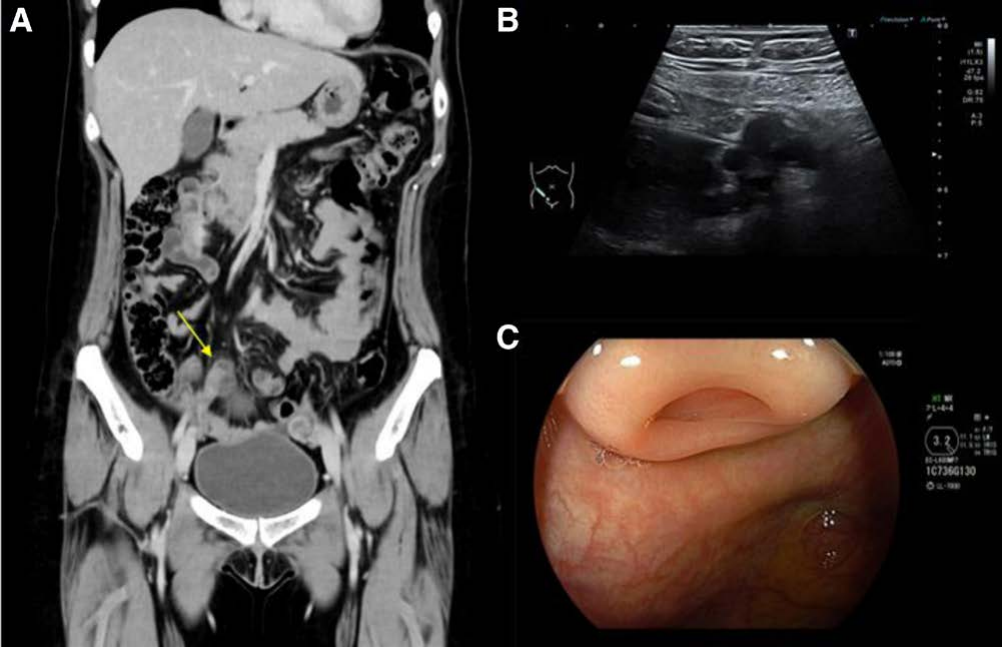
Lesion visualization. (A) Computed tomography (yellow arrow) and (B) ultrasonography of the abdomen showing a cystic lesion at the appendiceal distal end. (C) Colonoscopy showing a submucosal tumor-like elevation at the appendiceal orifice.
